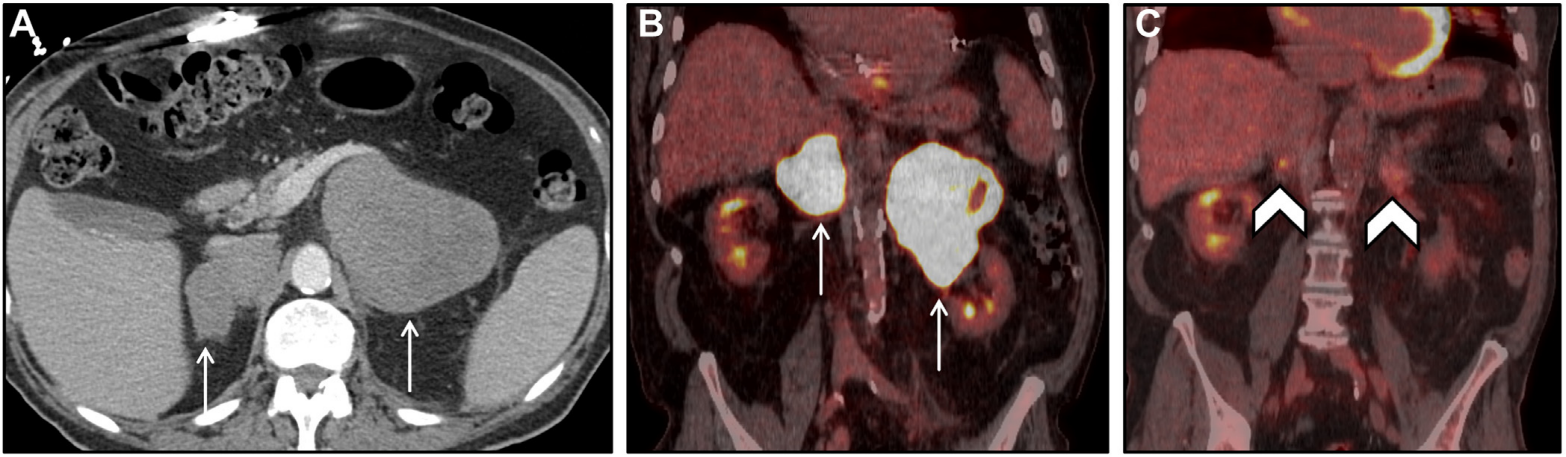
Bilateral adrenal lymphoma. (*A*) Axial CT image with contrast demonstrates large bilateral adrenal masses in a patient with diffuse large B cell lymphoma. (*B*) Fused coronal FDG PET/CT image demonstrates marked FDG uptake. (*C*) Follow-up fused coronal PET/CT image after treatment demonstrates interval decrease in size and FDG uptake of the bilateral lesions (arrow heads) in keeping with favorable treatment response. *CT* = computed tomography; *FDG PET* = fluorodeoxyglucose positron emission tomography.
